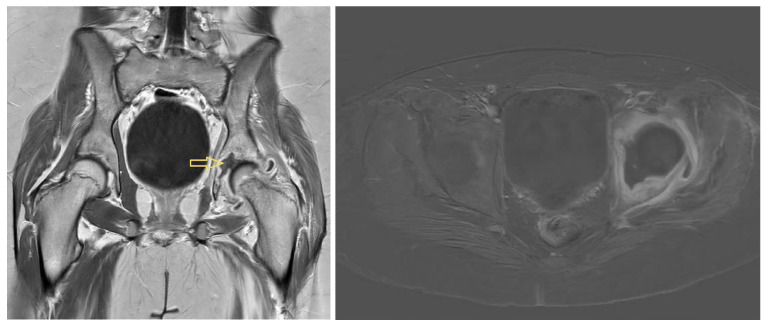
Magnetic resonance images of Patient 3 on hospitalisation day 40: osteomyelitis in the left acetabulum with an abscess in the region of the former Y-joint. Arrow shows to the fluid path.
